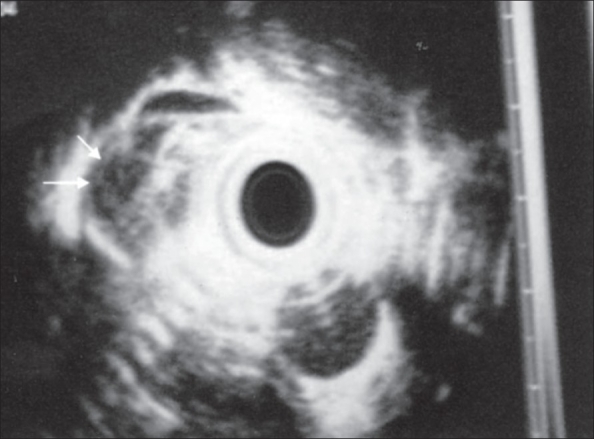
Endoscopic ultrasound showing a large sub-carinal lymph node with central necrosis and submucosal thickening with a large paraesophageal lymph node adherent to the esophagus near gastroesophageal junction
